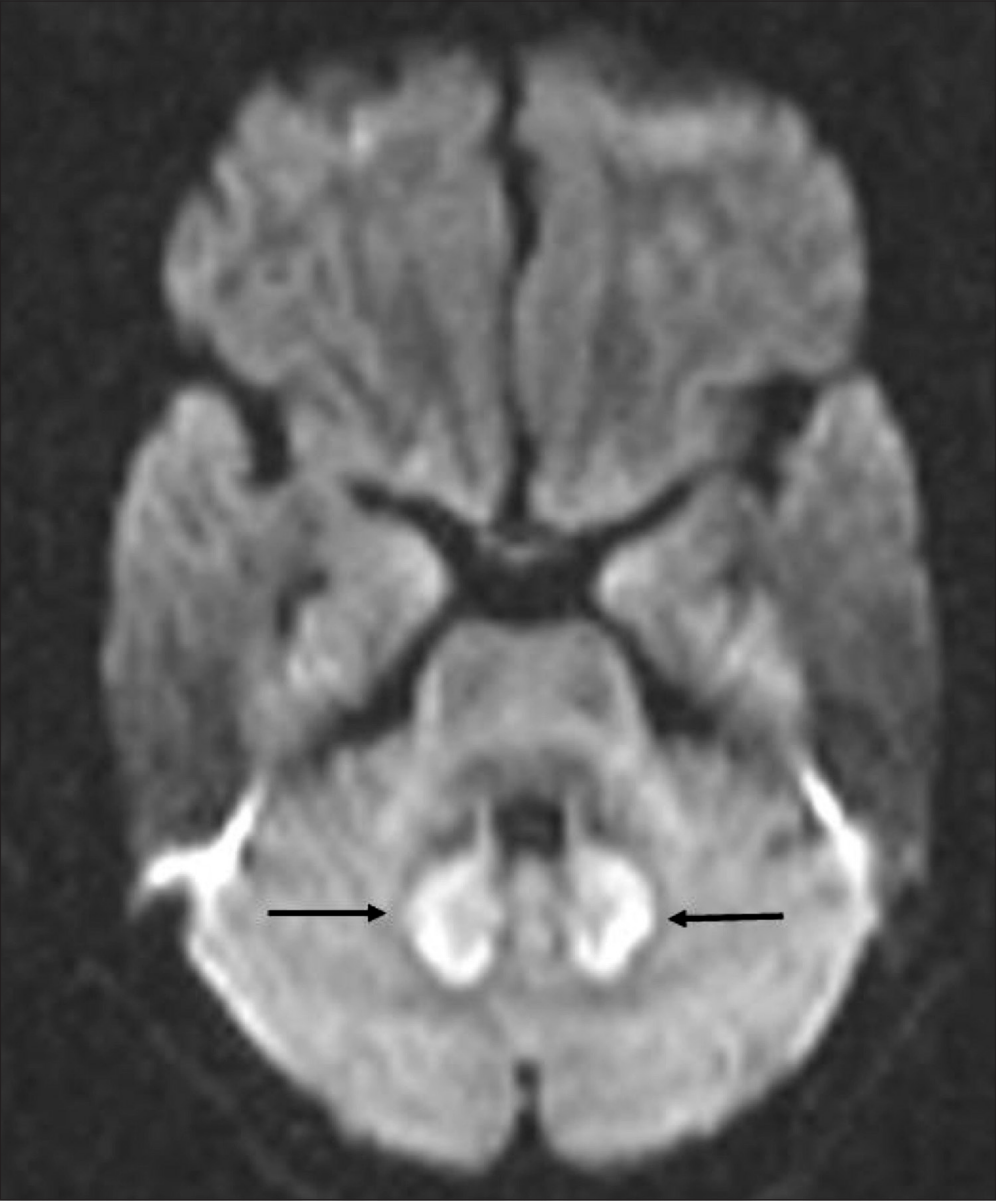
Axial diffusion weighted MRI image shows diffusion restriction in the dentate nuclei (arrow) and dorsal pons (arrowhead)
